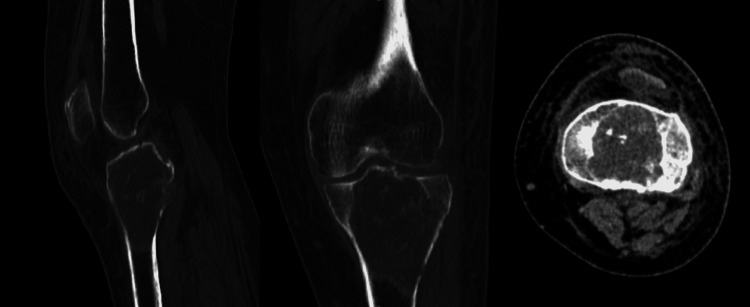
CT scan suggests a lytic lesion in the proximal tibia with areas of cortical erosion
